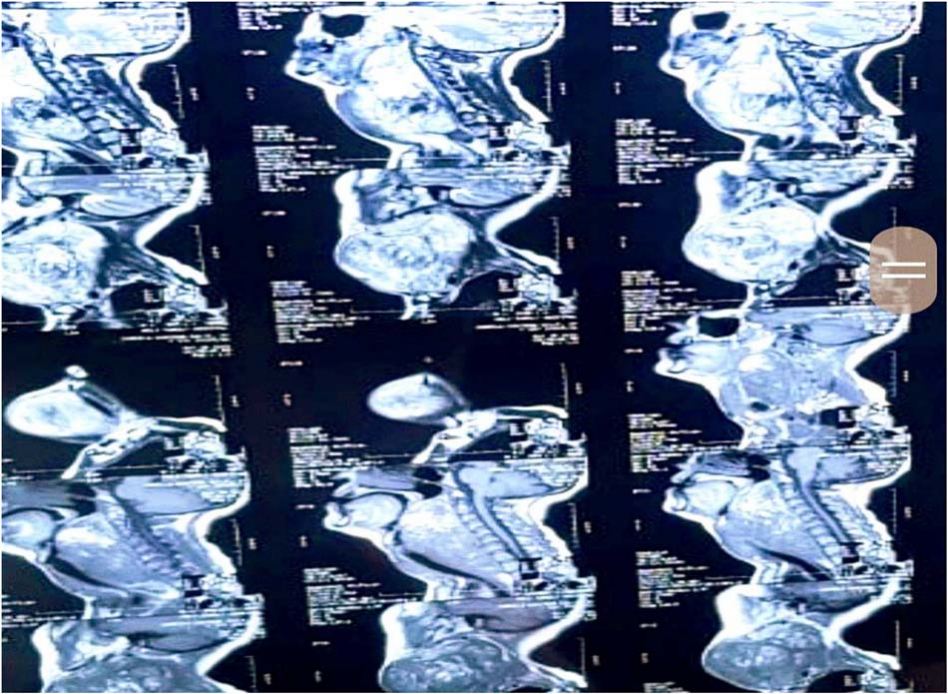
MRI scan demonstration of mass location and extension depicted at various levels of scan measuring 10 × 13.5 × 12.5 cm in size and appearing heterogeneously isointense on T1W1 scan and heterogeneously hyperintense on T1WI and STIR sequence.
